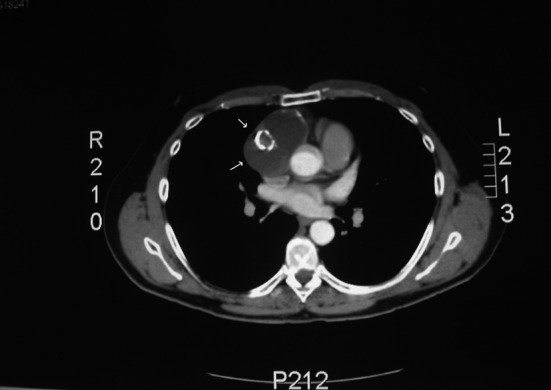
Chest computed tomography (axial view) with cystic lesion in the pericardium (arrows).
